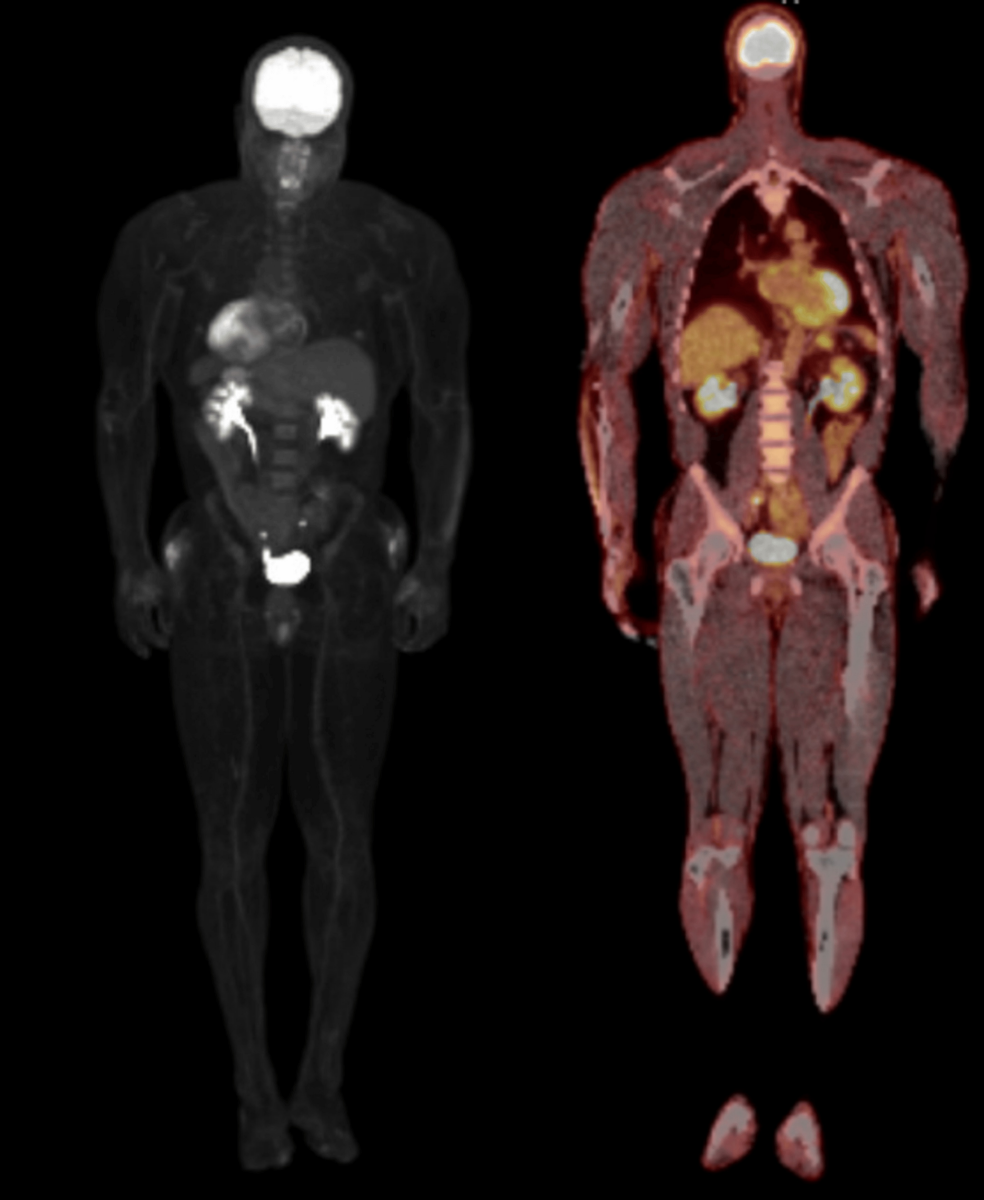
PET-CT showing no abnormal uptake
